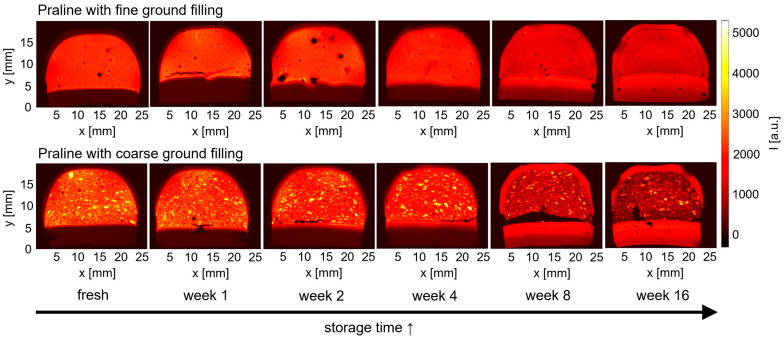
MR images of pralines with a fine ground (praline 1, top) and coarse ground (praline 12, bottom) filling in the longitudinal study (storage at 20 °C). The pralines were removed from storage and measured after storage times of 1, 2, 4, 8 and 16 weeks. Between the fourth and eighth week in particular, the signal intensity of the chocolate shell increases, while it decreases for the filling.
